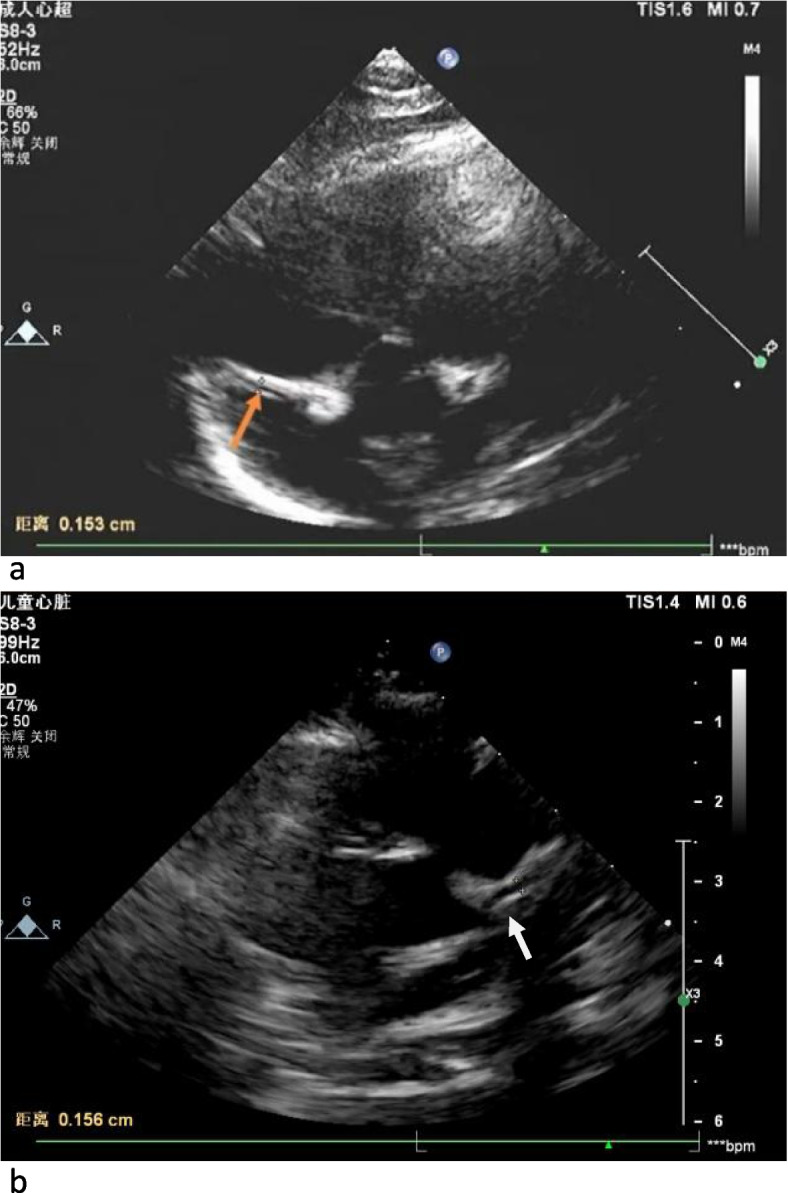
Echocardiography on the 46th day of disease course. Ultrasound examination of the heart showed that coronary artery dilation was improved. a Five chamber view showing dimensions of right coronary artery. b Parasternal short axis view showing left coronary artery (4a and 4b)
